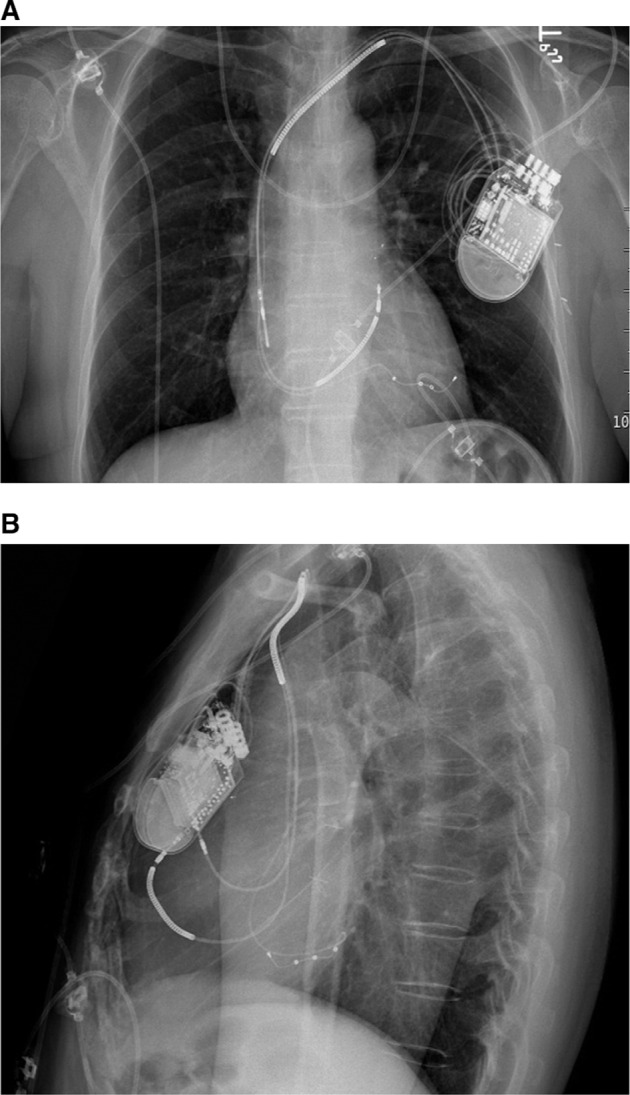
Posteroanterior (A) and lateral (B) chest X-rays demonstrating the ICD lead in the anteroseptal position that resulted in para-Hisian pacing.
